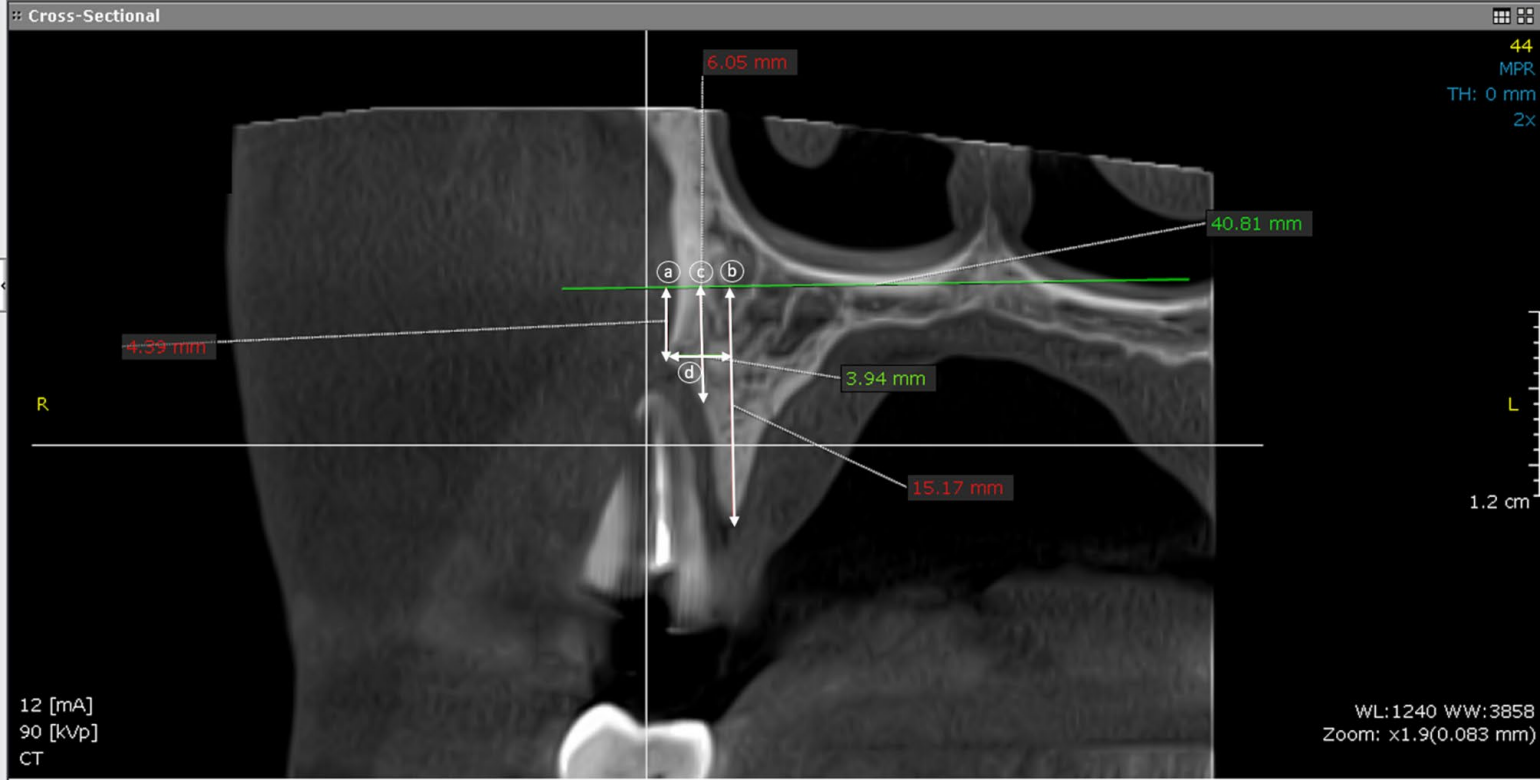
Radiographic measurement of bone width and height before surgery. (a) The measurement from the floor of the maxillary sinus to the top of the buccal alveolar bone (b) The measurement from the floor of the maxillary sinus to the top of the palatal alveolar bone (c) The measure from the floor of the maxillary sinus to the socket wall halfway between the palatal and buccal bony peaks. (d)The bone width is achieved by measuring the distance from points (a) to (b)
